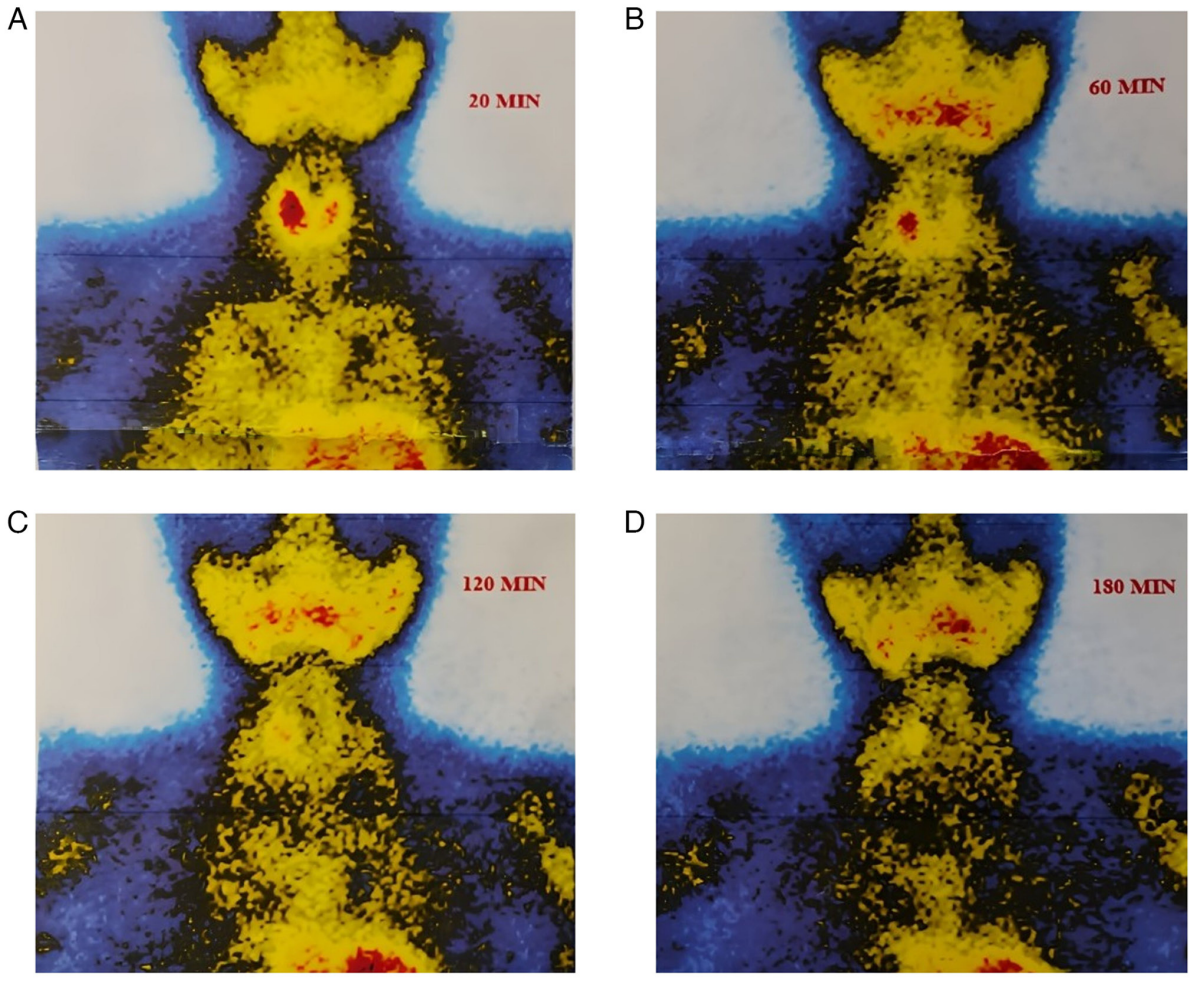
Sequential 99mTc-sestamibi scintigraphy images obtained at different time intervals demonstrating radiotracer uptake and washout patterns in the thyroid region. (A) At 20 min (early phase), there is intense radiotracer uptake in both thyroid lobes, with a focally increased accumulation in the right thyroid lobe, suggesting a hyperfunctioning lesion. (B) At 60 min, partial washout of tracer activity is observed from most of the thyroid tissue, while the right thyroid lobe shows persistent uptake. (C) At 120 min, further washout from normal thyroid tissue occurs, with sustained focal uptake in the right lobe. (D) At 180 min, complete washout from the thyroid gland is seen, except for persistent radiotracer retention in the mid-portion of the right lobe, confirming the presence of an intrathyroidal parathyroid adenoma.
